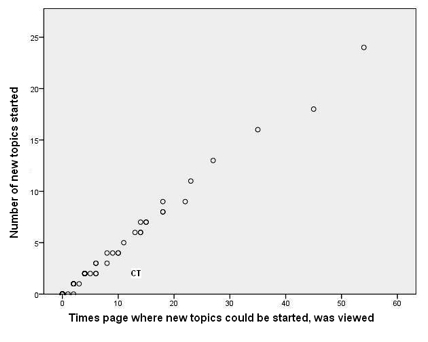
Detail from Figure 3, showing Caretaker (CT) as outlier, having viewed the page from were new topics are started many more times than she or he started new topics
